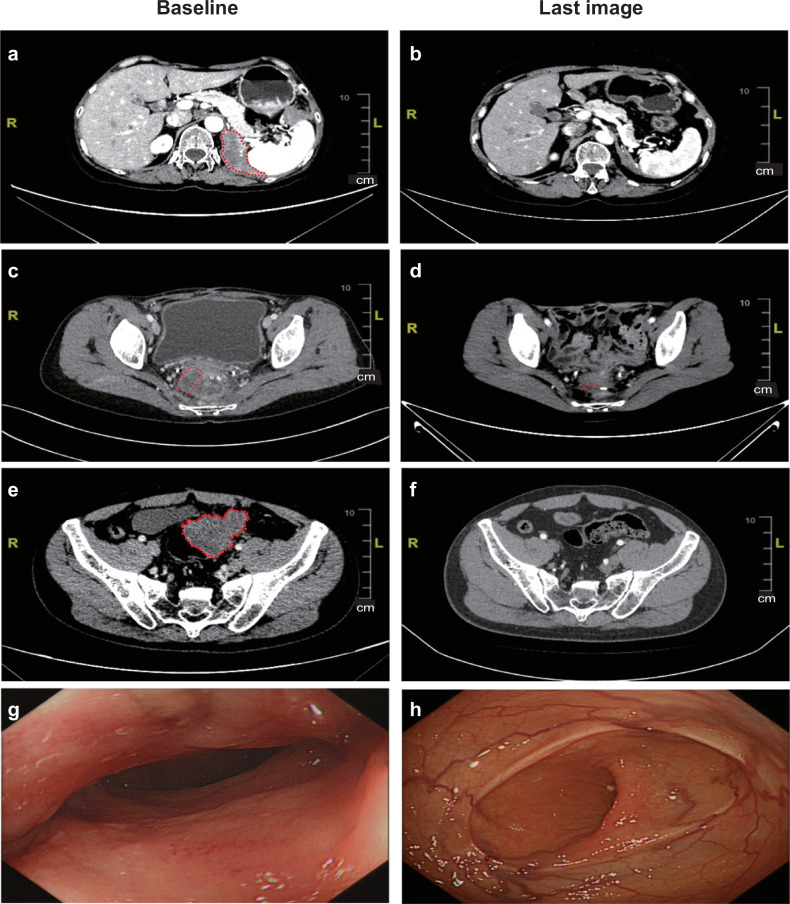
Objective tumor responses in three patients with metastatic solid tumor. CT scan showing peritoneal metastasis and its evolution between baseline (a) and after toripalimab (b) of patient SYSUCC07 with metastatic renal medullary carcinoma harboring R682Q germline *POLD1* mutation. c, d The regression of the peritoneal metastasis of patient SYSUCC09 diagnosed with colon cancer harboring S297F and S1906Y *POLE* mutation. e, f The locally recurrent primary tumor and its evolution between pretreatment and after toripalimab in patient SYSUCC10 diagnosed with colon cancer harboring P286R and F1907L *POLE* mutation, who achieved complete response. g, h The colonoscopy image at baseline and after treatment of patient SYSUCC10, respectively
